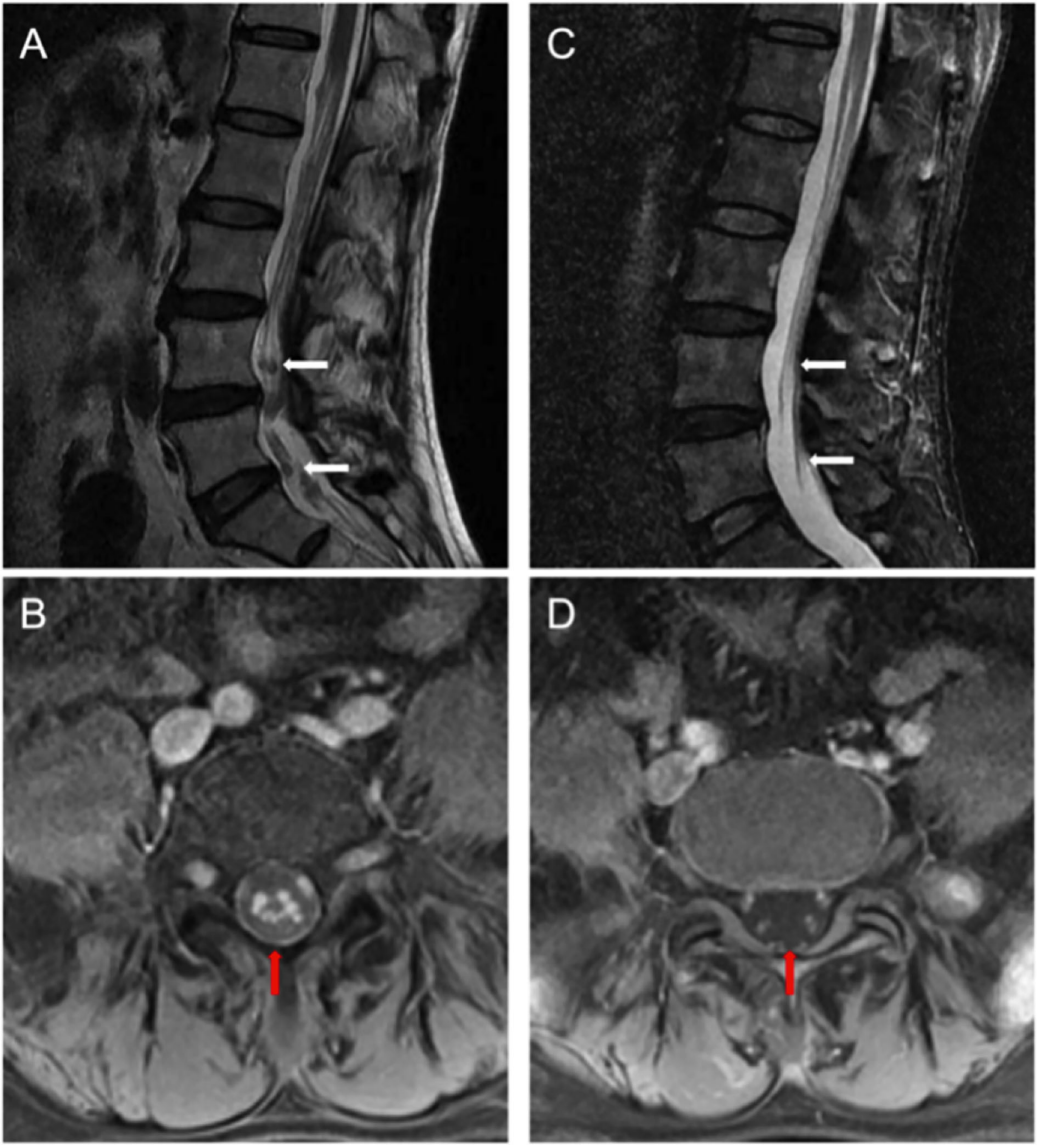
(A) Pretreatment lumbar MRI showed multiple diffuse abnormal signal nods in the cauda equina at lumbar and caudal level. (B) Enhanced transversal scanning. (C) Lesion disappeared in the post-treatment imaging, indicating a complete remission (CR). (D) Enhanced transversal scanning also verified complete remission of these lesions after BCMA CAR-T cell therapy [high-resolution T2-weighted image].
